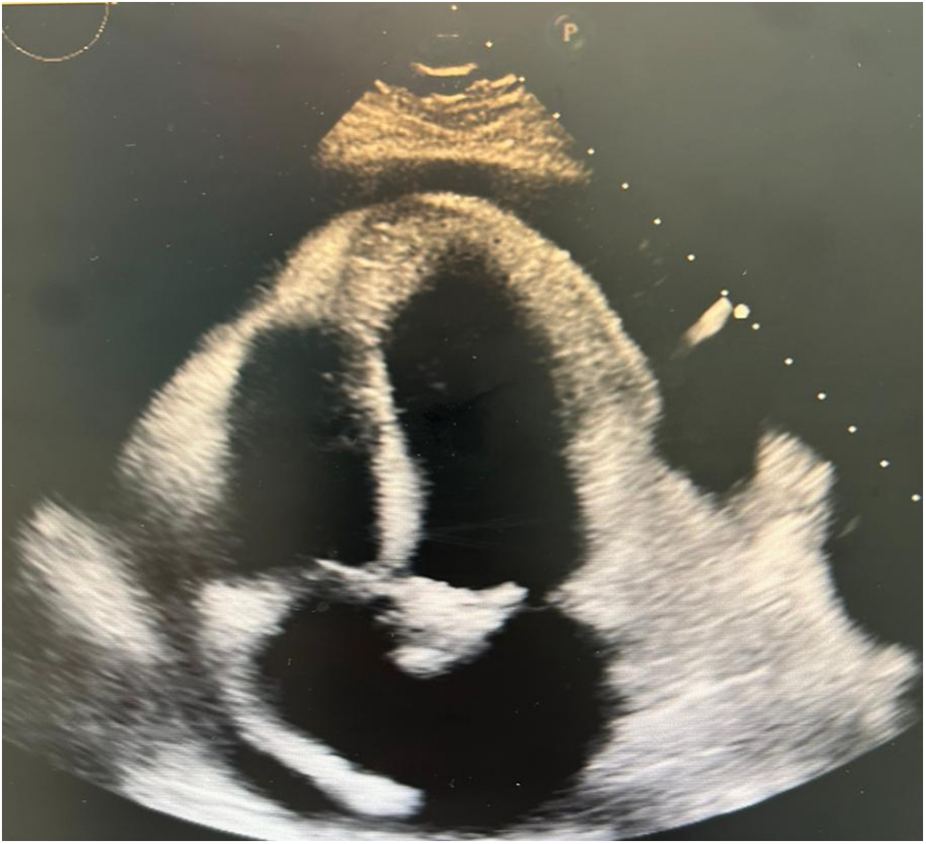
Echocardiogram 2D showing pericardial effusion, no signs of tamponade.
